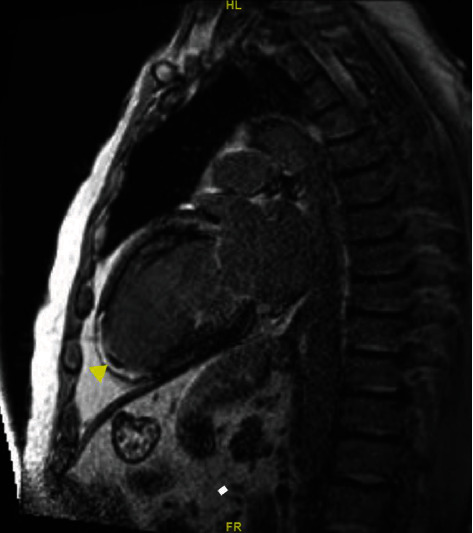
Delayed enhanced cardiac magnetic resonance image. Delayed enhanced cardiac MR (CMR) demonstrating an apical laminar thrombus (blocked arrow).
